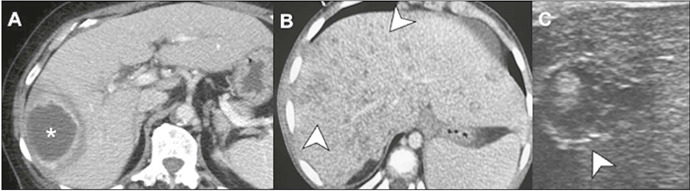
Specific liver infections. Contrast-enhanced CT scan
